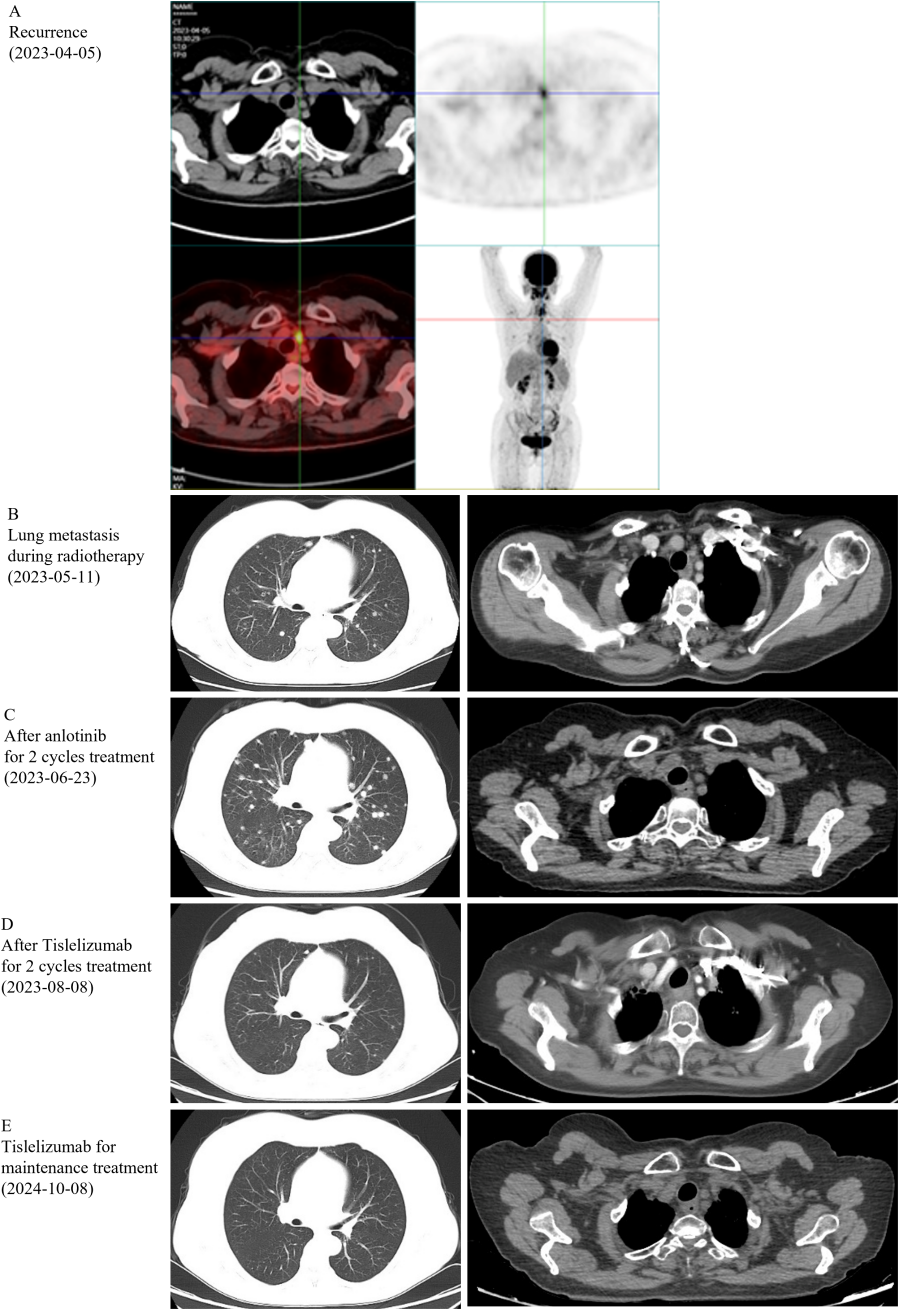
Imaging results. (A) PET-CT was performed on the recurrence, on Apr 5, 2023. (B-E) Computerized tomography (CT) was performed after different stages of treatment.
